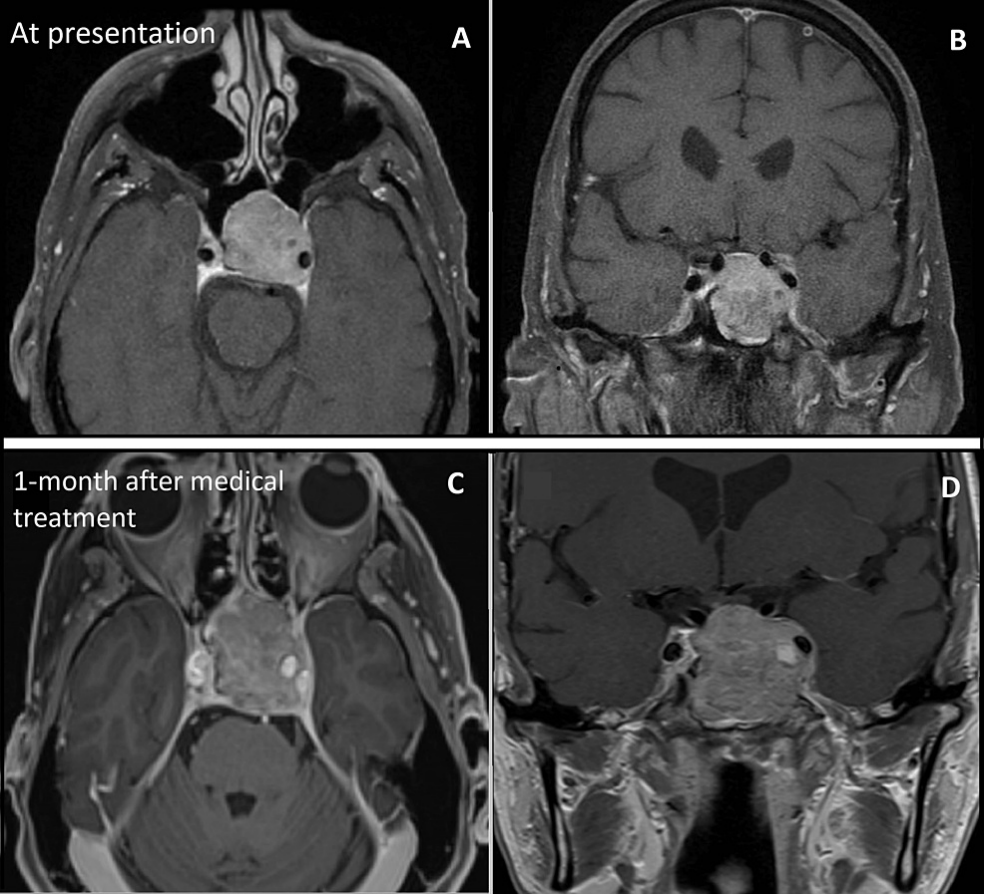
Imaging at the time of consultation and after one month of medical treatment A, B: Axial (A) and coronal (B) T1-weighted gadolinium-enhanced MRI showing the parasellar lesion when the patient consulted for his visual symptoms. The lesion substantially grew over the years. C, D: Axial (C) and coronal (D) MRI sequences show the abnormally rapid growth of the lesion one month after the medical treatment was started MRI: magnetic resonance imaging
